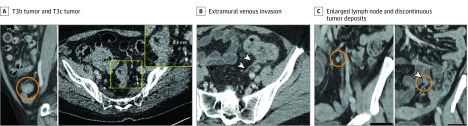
T Stage, N Stage, and Extramural Venous Invasion on Computed Tomography A, T3b tumor (within circle) shows 1- to 5-mm extramural spread; T3c tumor (inset) shows >5- to 15-mm extramural spread. B, Extramural venous invasion (arrowheads) from a tumor along the length of a vein. C, Enlarged lymph node (circle in left image) vs discontinuous tumor deposits (circle and arrowhead in right image) along the course of a vein.
